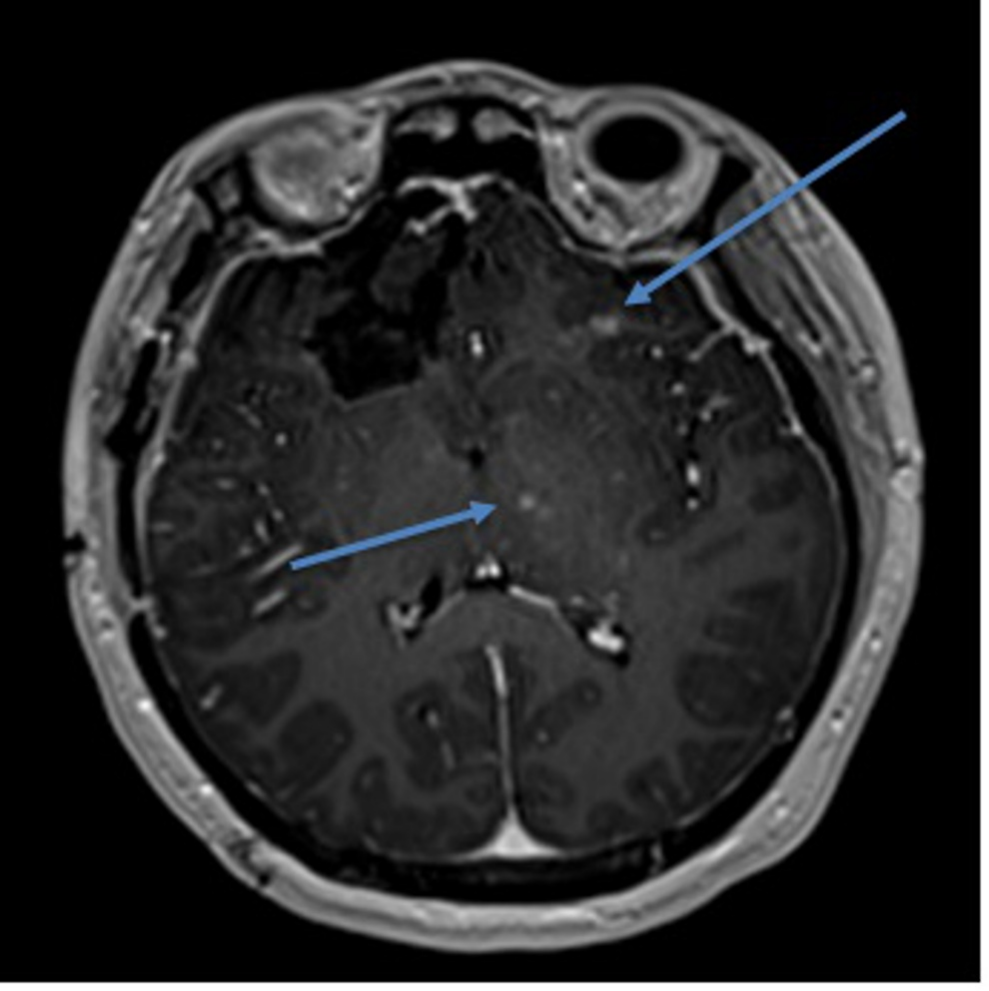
T1-weighted brain magnetic resonance imaging with contrast Arrows indicate areas of enhancement distant from the surgical field.
